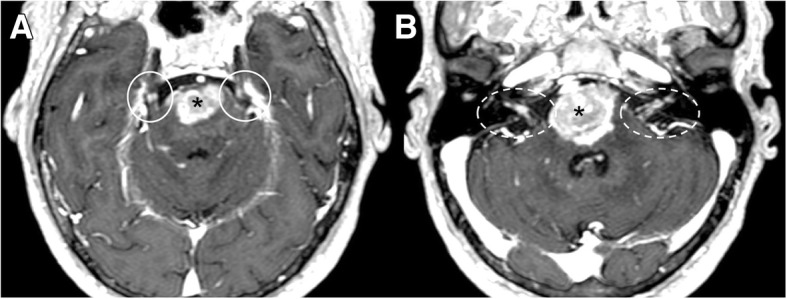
Leptomeningeal spread of tumor. Patient with lung carcinoma. MRI axial image of T1-weighted post-gadolinium sequence demonstrates a large metastasis of the pons (asterisks), bilateral leptomeningeal enhancement of trigeminal nerves (a, circles), and bilateral facial and vestibulocochlear nerves (b, dotted circles)
